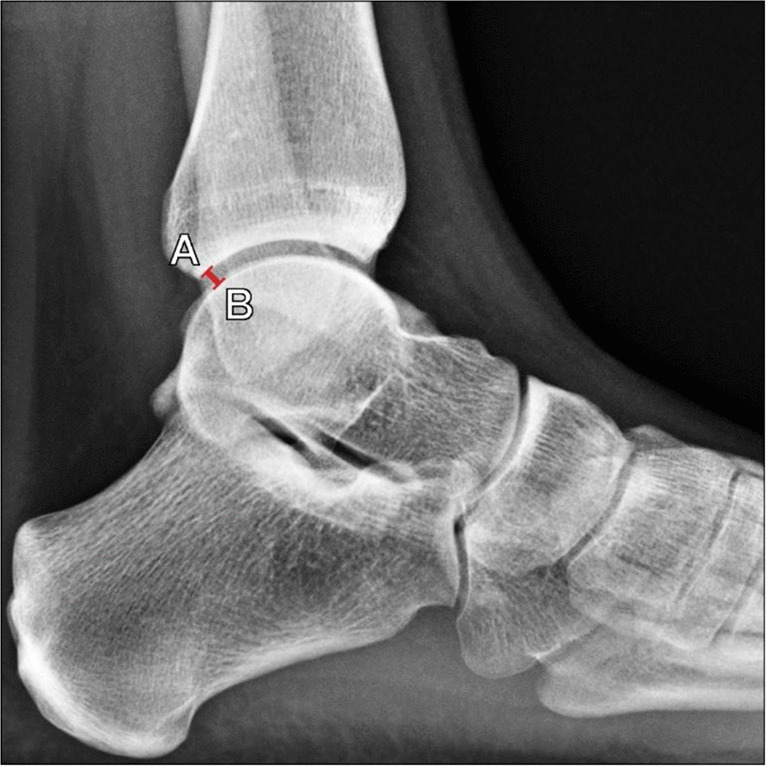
Measurement of the anterior talar translation on a lateral weightbearing radiograph of the ankle. The most reliable method for evaluation of anterior talar translation measures the shortest distance between the posterior lip of the tibial plafond (A) and the posterior talar dome articular surface (B). Normal values in neutral position are < 4 mm, and < 6 mm with anterior drawer stress. An increased anterior talar translation distance indicates anterior talofibular ligament insufficiency
